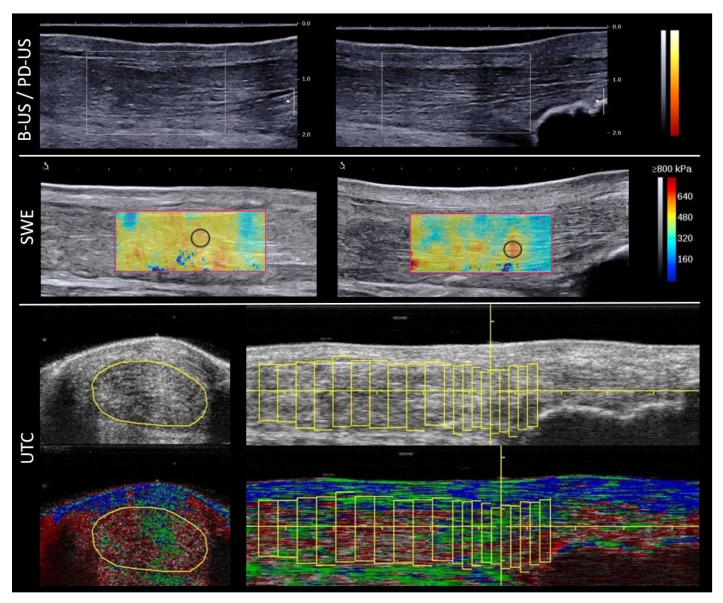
B-Mode (B-US), Power Doppler (PD-US), Shear Wave Elastography (SWE) and Ultrasound Tissue Characterization (UTC): asymptomatic patient 12 months after midportion rupture and suture. There is no neovascularization, but focal hypoechogenicity and a thickened tendon in B-US and PD-US. For the insertion, SWE revealed high elastic properties (SWE 3 mm (black circle): 538.4 kPa (13.38 m/s) SD 12.3 kPa (2.02. m/s); SWE area (red rectangle): 469.5 kPa (12.49 m/s) SD 78.3 kPa (5.1 m/s), area 2.44 cm2). UTC shows a large area of echo type III (red—fibrillar matrix) in the midportion in the axial and longitudinal planes. UTC echo type I—intact and aligned tendon bundles (green); UTC echo type II—discontinuous wavy tendon bundles (blue); UTC echo type III—mainly fibrillar matrix (red); UTC echo type IV—mainly amorphous matrix (black).
